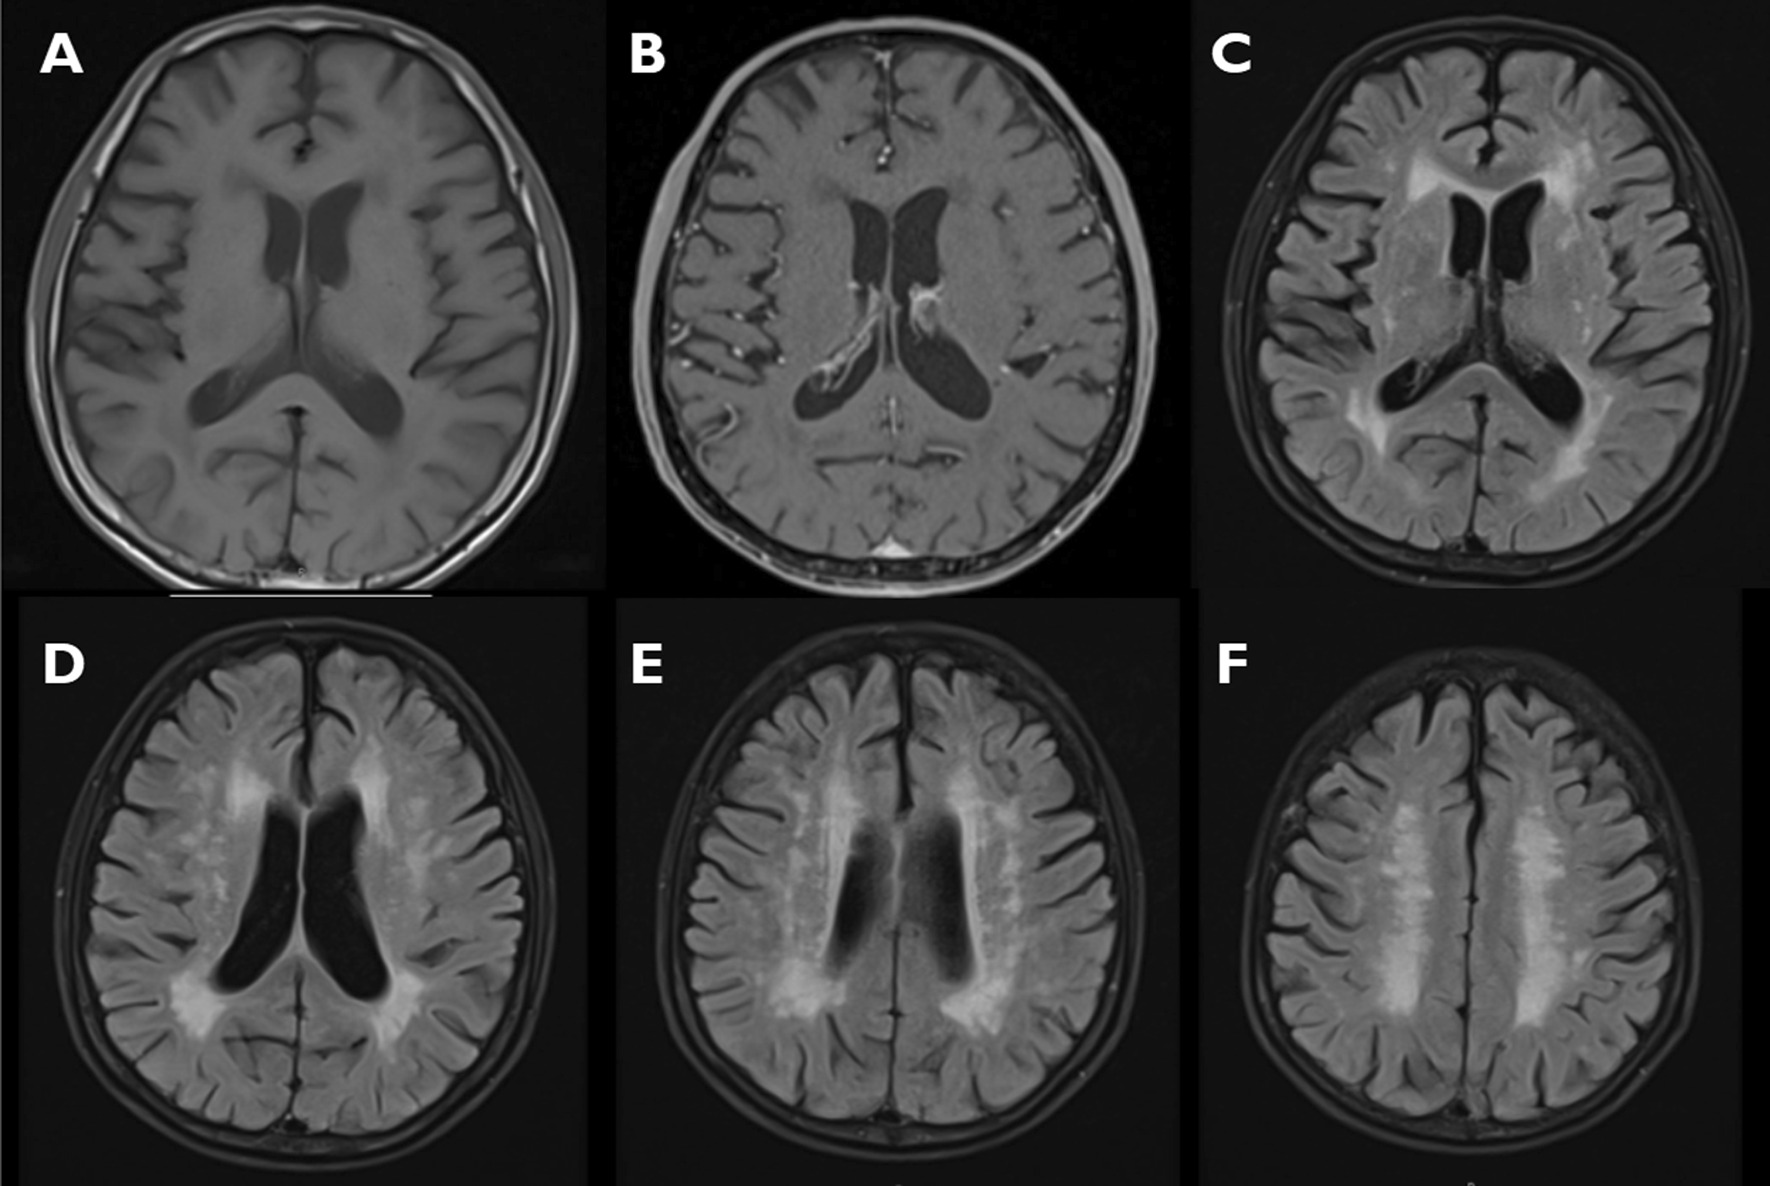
MRI brain T1-weighted sequence (A), T1-weighted sequence with gadolinium (B), T2-fluid-attenuated inversion recovery (C–F). MRI was performed 2 days after the second course of mFOLFOX6. The study on T2 fluid-attenuated inversion recovery demonstrated multiple nonspecific periventricular and deep subcortical white matter changes in the bilateral cerebral hemispheres, as well as internal and external capsules. Mild cerebral volume loss is noted. No space-taking lesion, restricted diffusion, or abnormal enhancement on gadolinium study
